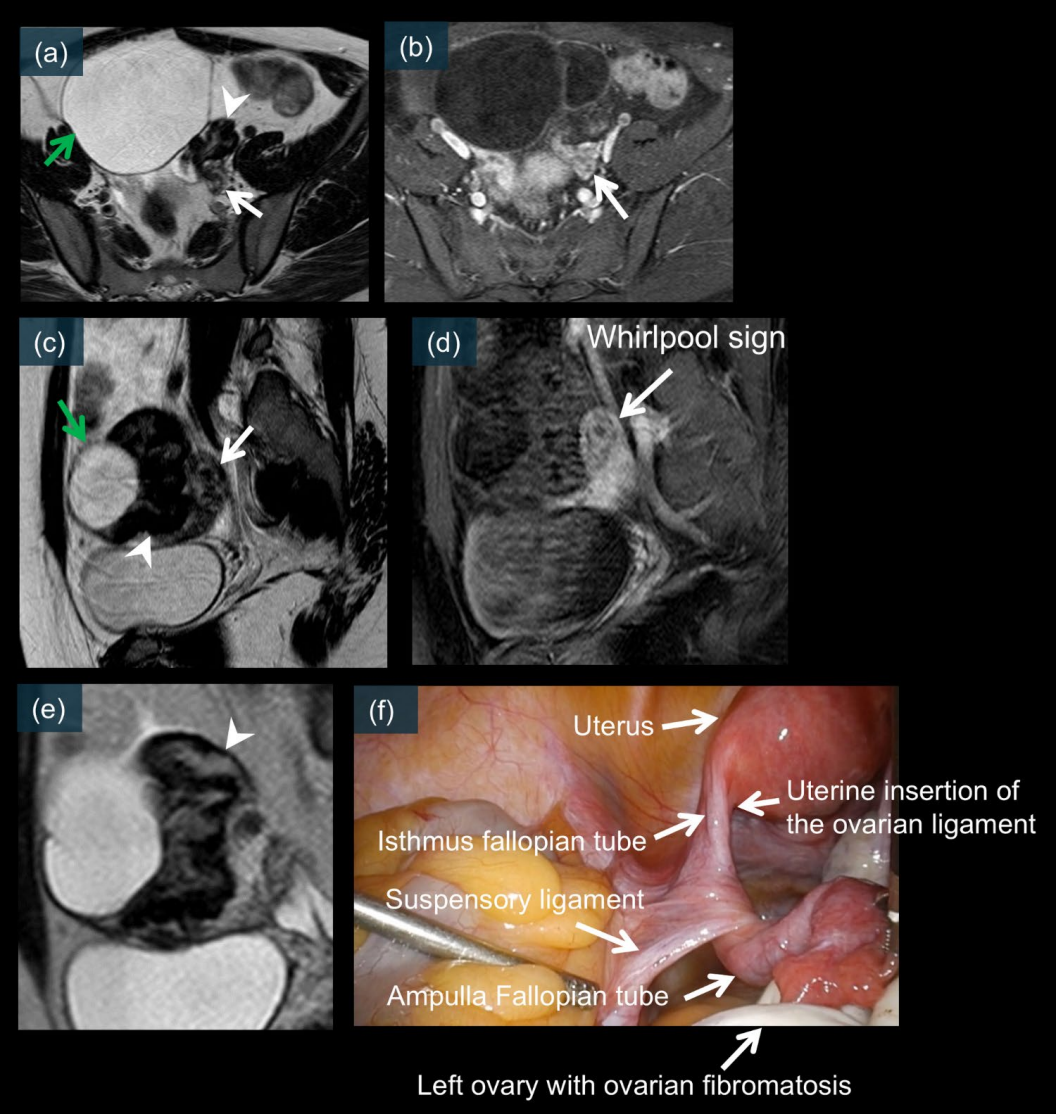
A 29-year-old female. a–d) show pelvic MR images. On T2WI (a and c), a multilocular cystic mass is observed in the left ovary (green arrows). Furthermore, a component with low signal intensity at the periphery on T2WI is noted (a, c and e, white arrowheads), which is considered to be the black garland sign. A twisted pedicle is also seen contiguous with this structure (a–c, white arrows). A whirlpool sign is clearly visible on the contrast-enhanced fat-suppressed T1WI sagittal image (d). f is a laparoscopic image, which reveals a 540° torsion of the left ovarian mucinous cystadenoma
